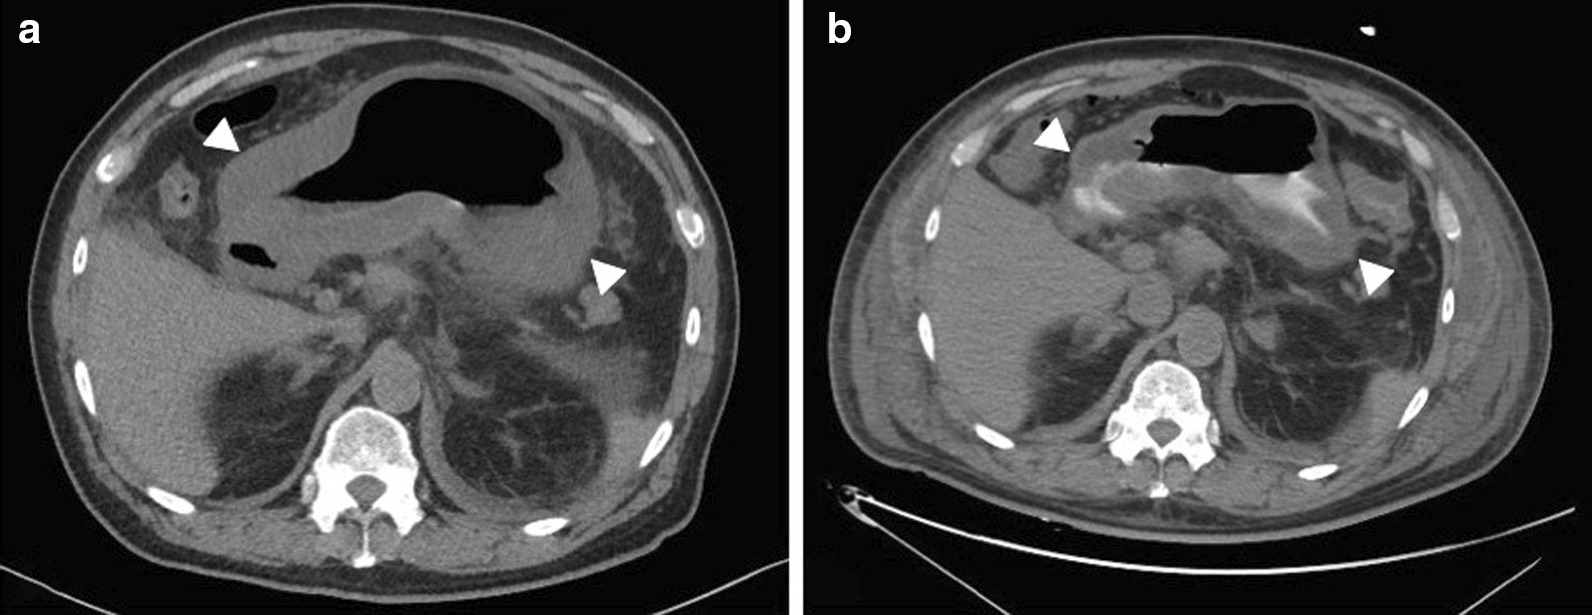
Computed tomography (CT). Axial view of the abdomen. a Typical findings of phlegmonous gastritis with diffuse gastric wall thickening (arrows), most pronounced distally. b Post-laparotomy demonstrates persistent diffuse gastric wall thickening (arrows)
